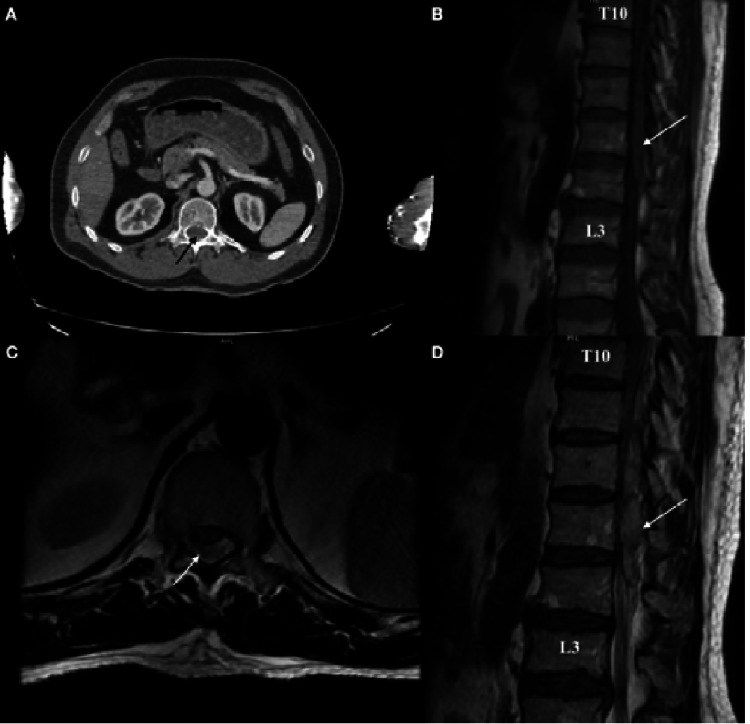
Preoperative images of the patient. A. CT image of isodense epidural hematoma located posterior to the spinal canal. B. T1-weighted sagittal MRI of iso/hypointense spinal epidural hemorrhage between the T10 and L2 levels. C. T2-weighted axial MRI of hyperintense spinal epidural hemorrhage located posterior to the spinal canal. D. T2-weighted axial MRI of hyperintense spinal epidural hemorrhage between the T10 and L2 levels. Arrows: spinal epidural hemorrhage.
